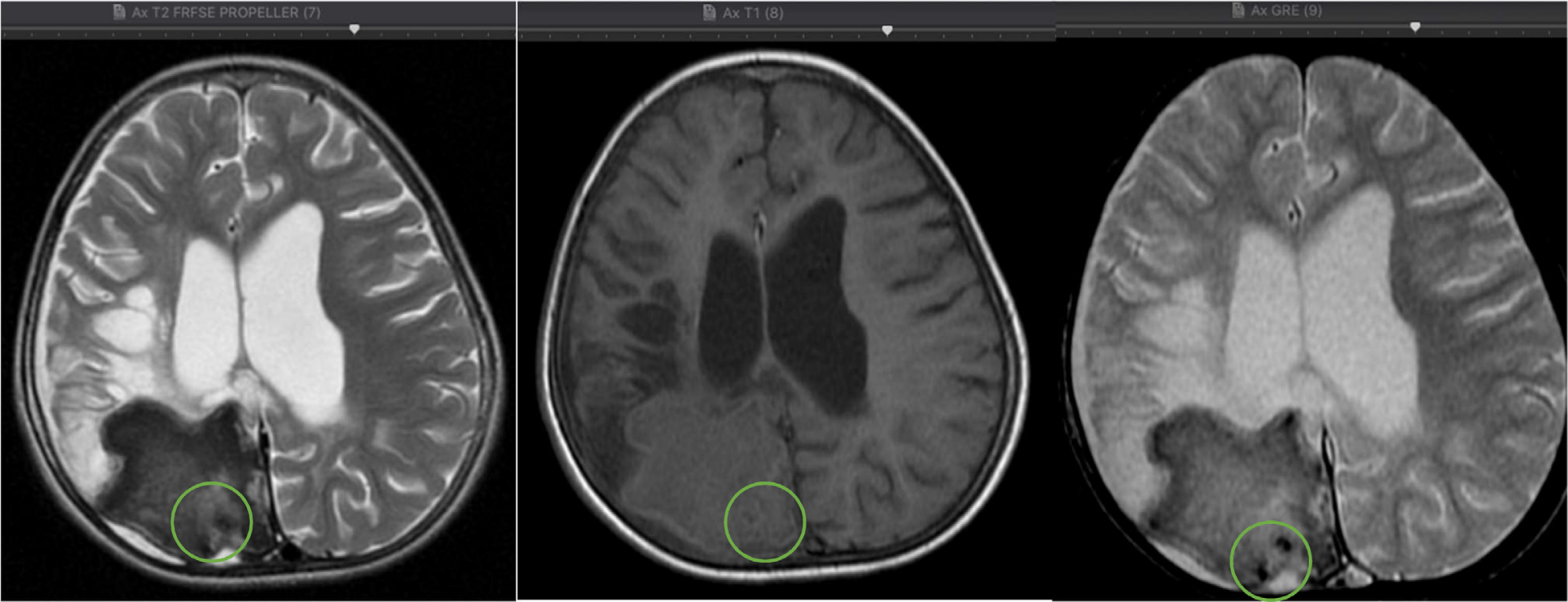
The axial section of the T1, T2 and GRE MRI, Multiple foci calcification in intra- and peri-cystic lesions in the cortex-subcortex of the right occipital lobe (on circle sign).
